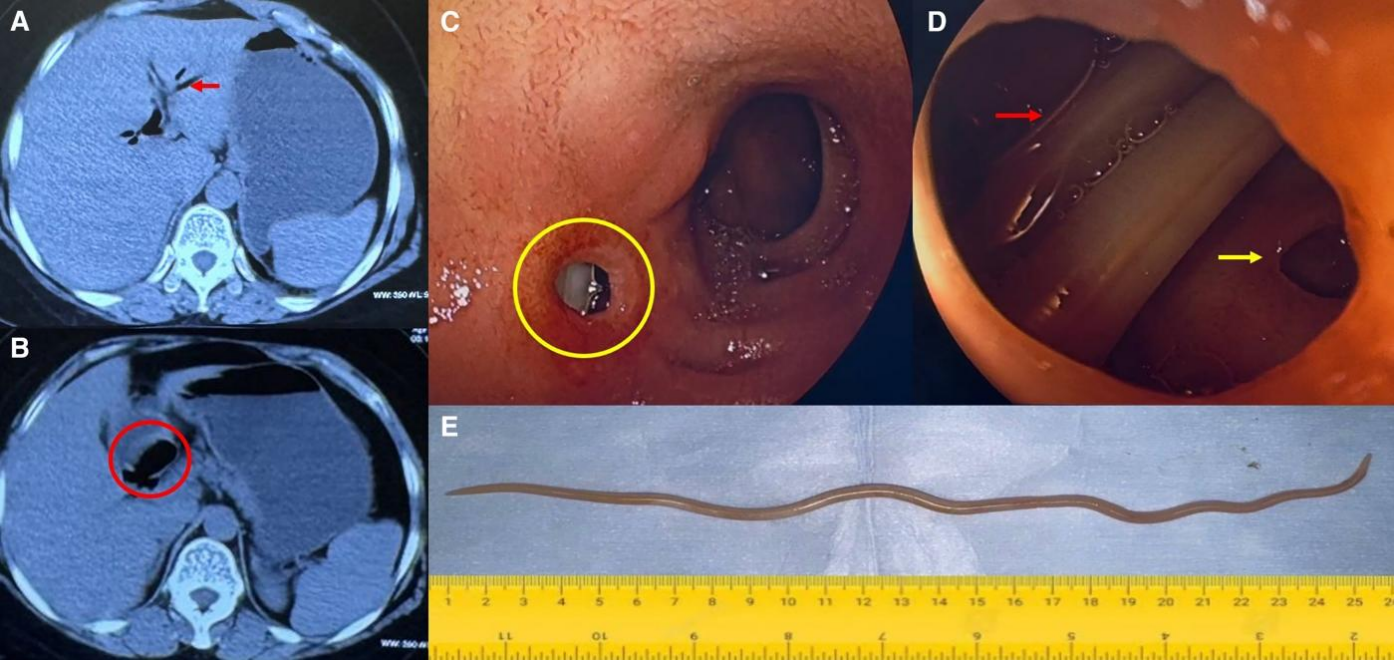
Computed tomography images (A) shows central and peripheral pneumobila (red arrow); (B) Air filled common bile duct adjacent first part of duodenum (red circle), suspicious of communication between them; (C) Upper Gastrointestinal endoscopy shows a fistulous opening in the anterior wall of the first part of the duodenum (yellow circle) with a worm inside the fistula; (D) Near view of the fistula shows *Ascaris lumbricoides* (red arrow) and biliary radicals (yellow arrow); (E) *Ascaris lumbricoides* retrieved from the fistula.
